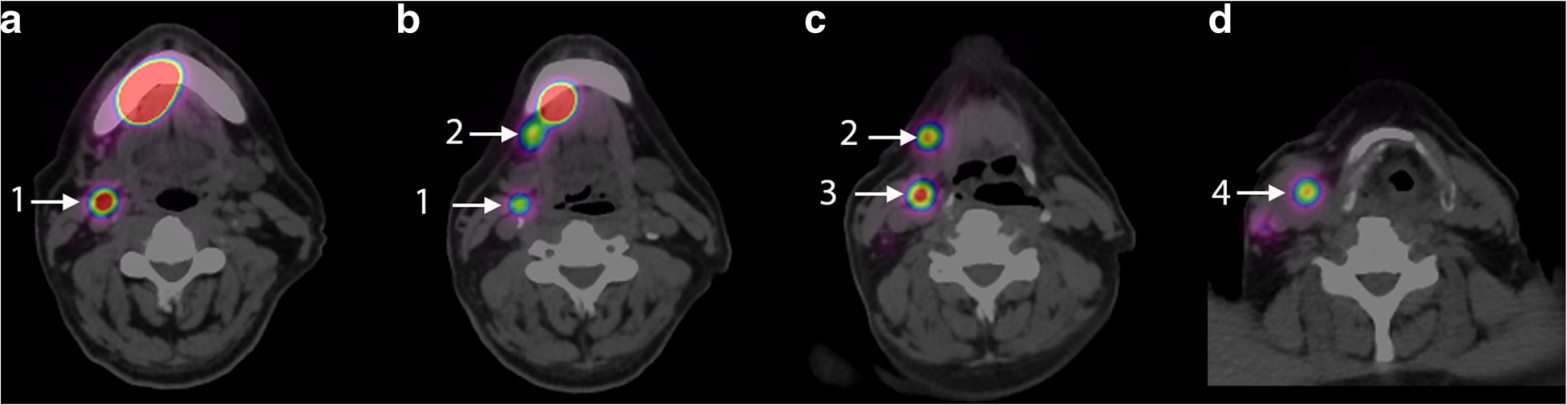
a–d Axial fused PET/CT images of patient 5 showing the injection site of ^89^Zr-nanocolloidal albumin in the floor of mouth on the right side and clear uptake in SLNs in levels IB (2), level II (1 and 3) and level III (4) on the right side. The distance between the injection site and the uptake in the SLN in level IB was 18 mm
